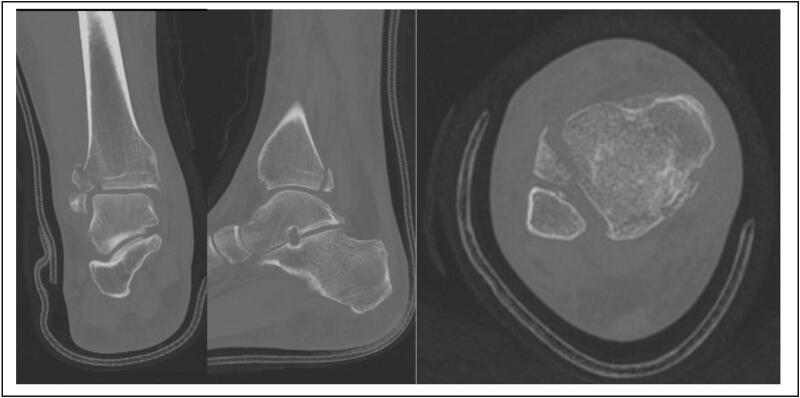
CT coronal, sagittal and axial views.
